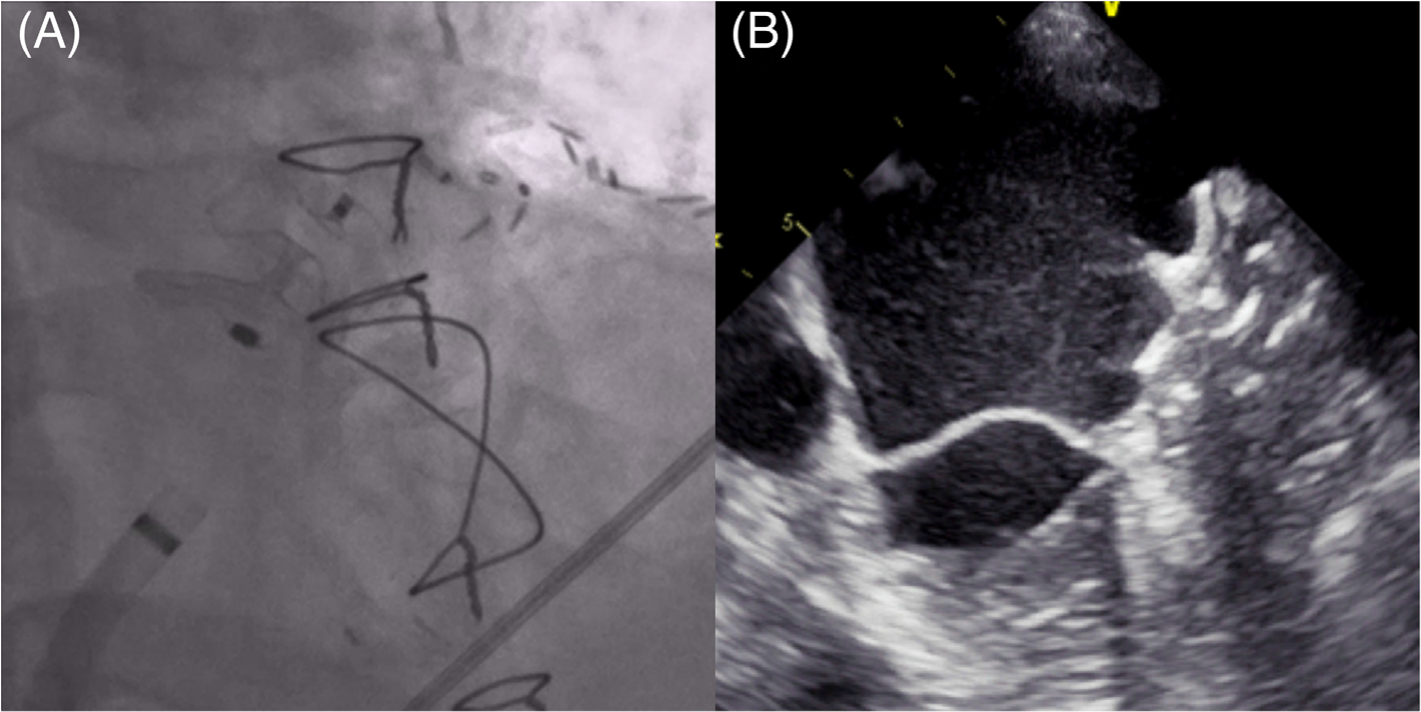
A, Fluoroscopic and B, echocardiographic image of the Amplatzer amulet device
